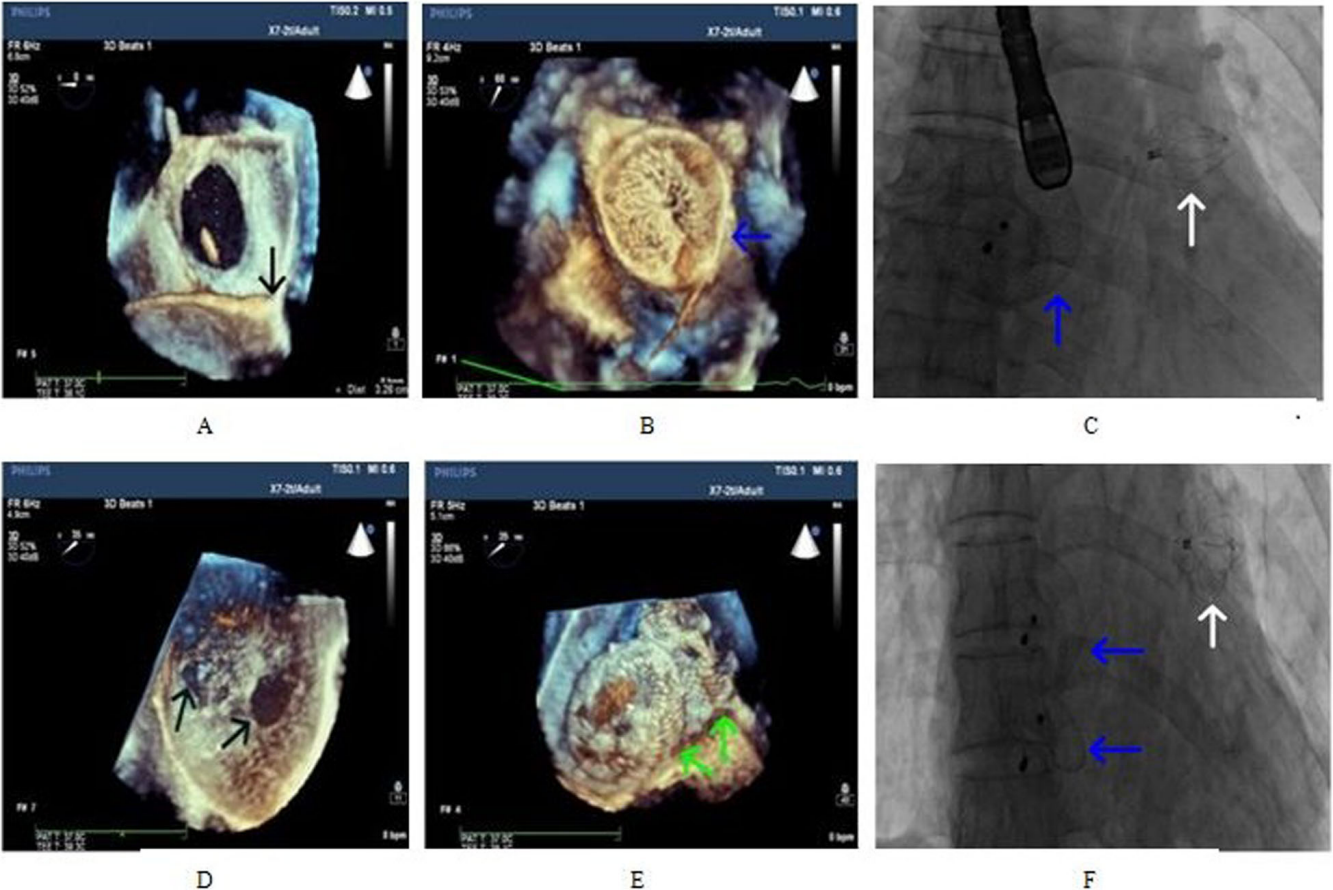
3D TEE imaging and X-ray imaging of successful LAAC and ASD occlusion for a large ASD patient and a double ASD patient with persistent AF (The black arrow indicates the LAA plug devices(a), dotted line indicates large ASD(a), blue arrow indicates the ASD plug devices(b). The blue arrow indicates the ASD plug devices, white arrow indicates the LAA plug devices(c); The black arrow indicates the double ASD(d), green arrow indicates the ASD plug devices(e); The blue arrow indicates the ASD plug devices, white arrow indicates theLAA plug devices(f))
